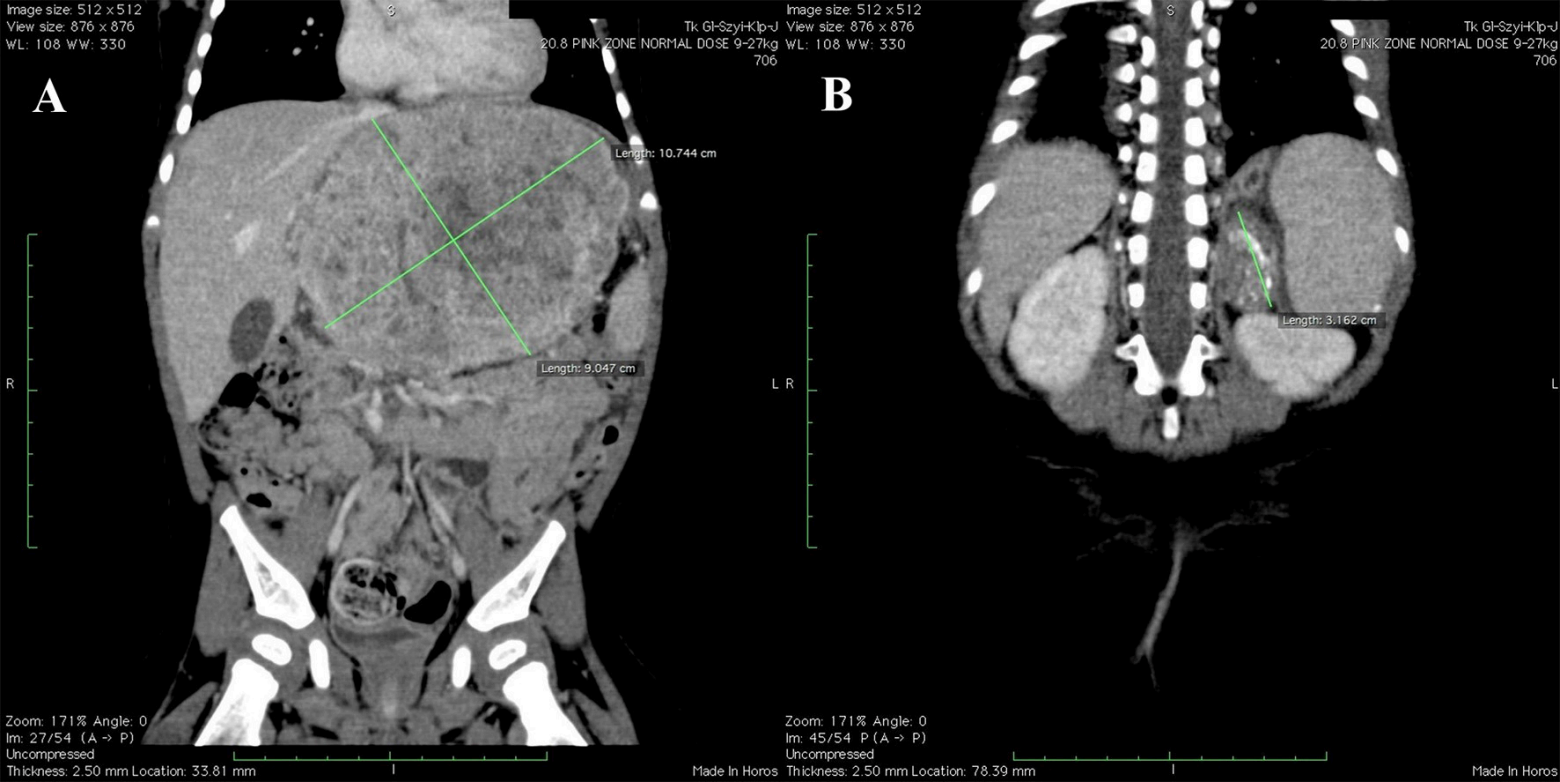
Initial CT scans. (A) Hepatoblastoma of the left hepatic lobe measuring 11 × 6.5 × 8.9 cm. (B) Neuroblastoma of the left adrenal gland measuring 2.3 × 1.6 × 3.6 cm.
